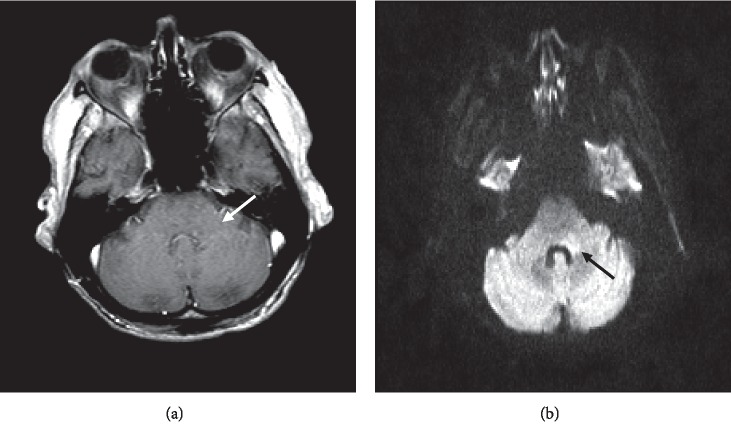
(a) T1 postcontrast image: lesion demonstrates lack of contrast enhancement. (b) Diffusion-weighted image (DWI) b1000: lesion demonstrates area of restricted diffusion at the level of the fourth ventricle involving the middle cerebellar peduncle.
